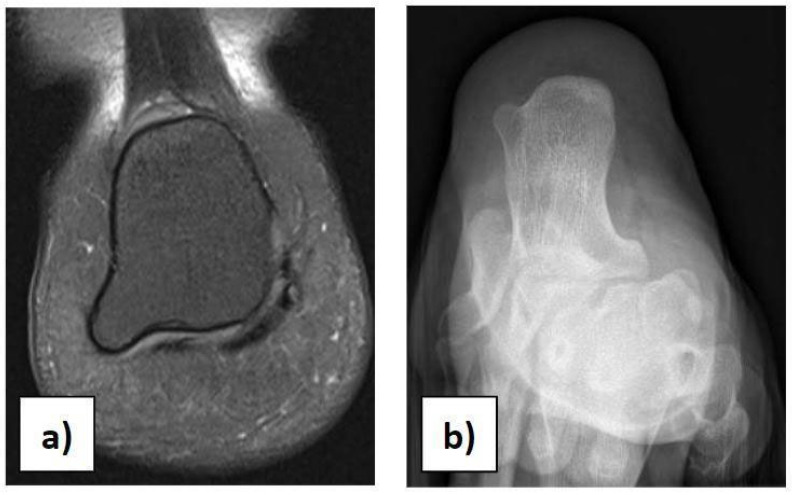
(a) Anatomical variation in the calcaneus on MRI. (b) Anatomical variation in the calcaneus on X-ray.
